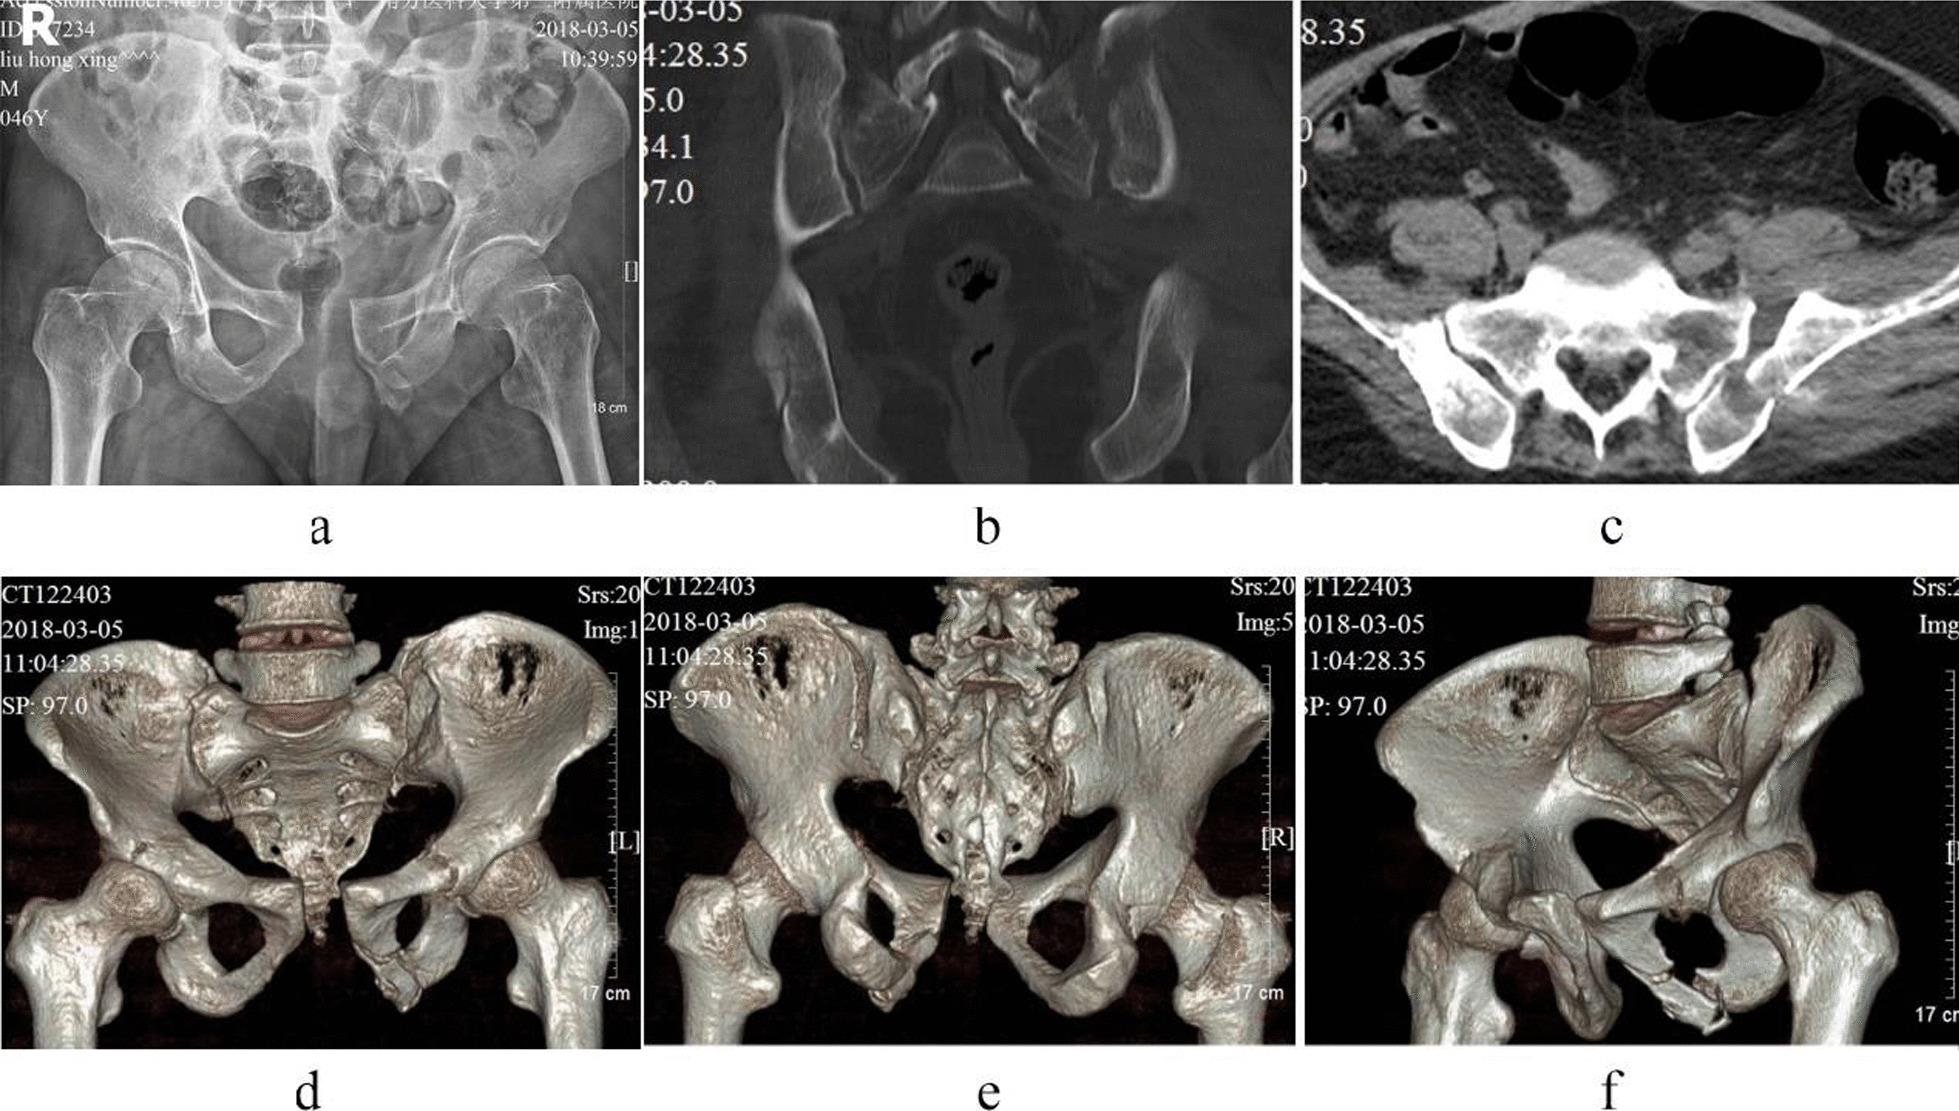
Preoperative images of case 3. (a Pelvic anteroposterior X-ray image, b CT sagittal image, c CT horizontal slice image, d–f CT 3D reconstruction images.)
